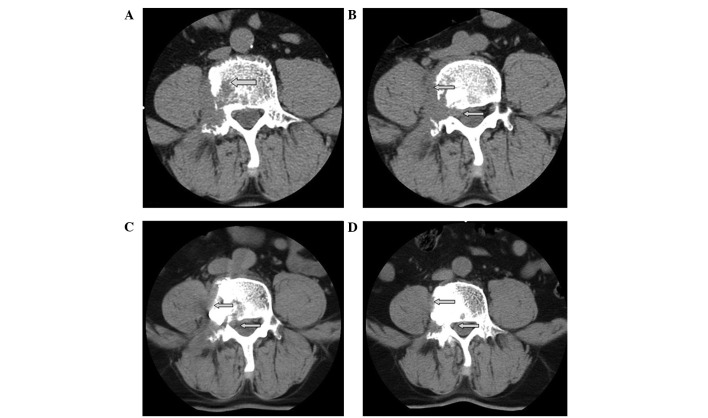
An osteolytic vertebral fracture in the right lateral mass of the L4 and accessories, accompanied by (A) a paravertebral and (B) intraspinal soft tissue mass. The mass (C) significantly decreased 5 days after PVP and (D, arrow) disappeared completely after 3 chemotherapy cycles. Computed tomography (CT) showed the distribution of bone cement in the osteolytic lesion of the L4 with minimal right lateral cement leakage. L4, fourth lower lumbar vertebra; PVP, percutaneous vertebroplasty.
